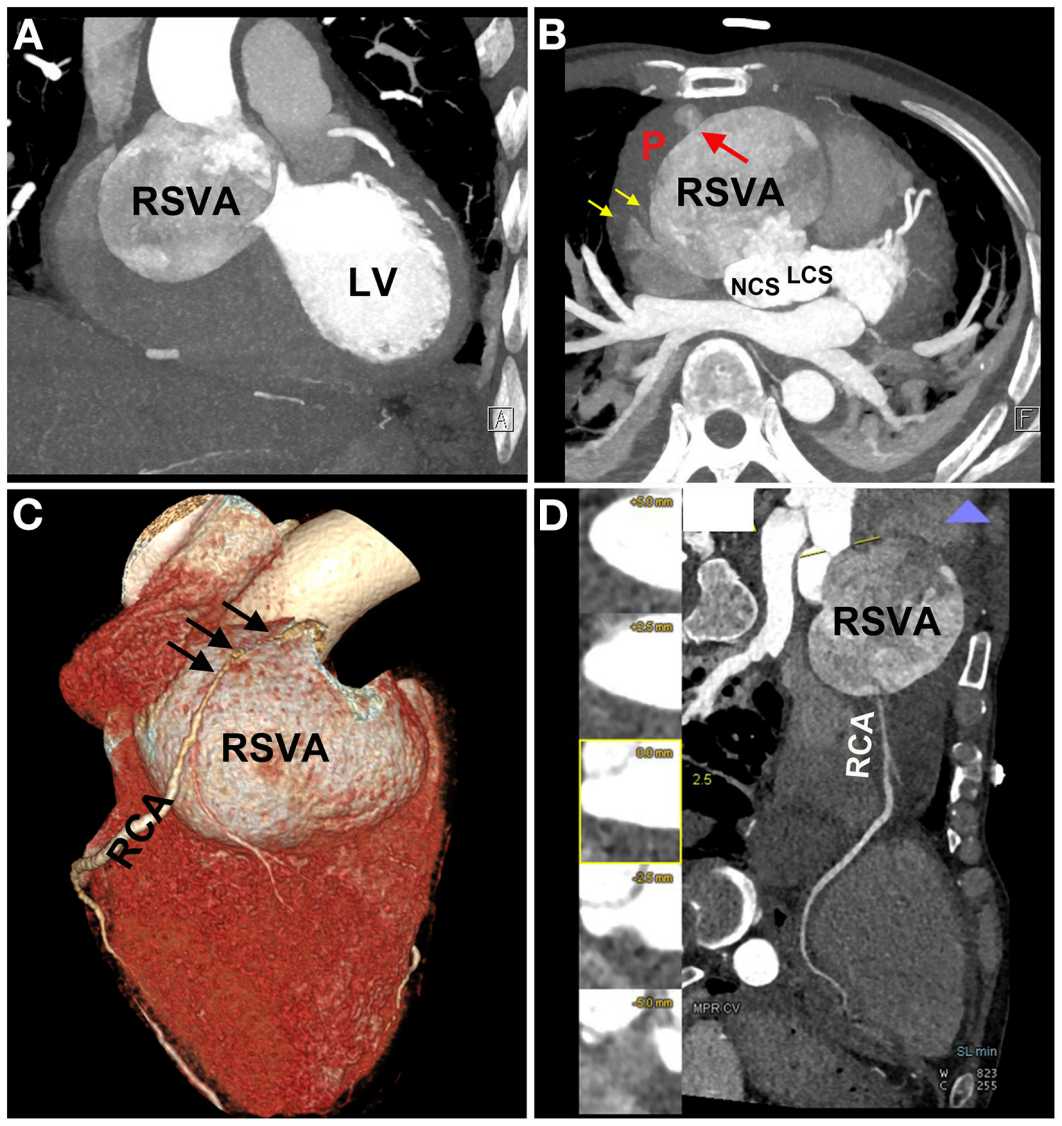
Coronary computed tomography angiography (CTA) images. CTA images showing the RSVA (A), which ruptured to the pericardium [red arrow (B)], and some contrast medium leakage into the pericardial sac [yellow arrows (B)]; the severely compressed RCA is stretched across the aneurysmal surface [black arrows (C)]; the proximal segment of RCA was relatively slender (D). RSVA, right sinus of Valsalva aneurysm; LV, left ventricle; NCS, noncoronary sinus; LCS, left coronary sinus; RCA, right coronary artery.
